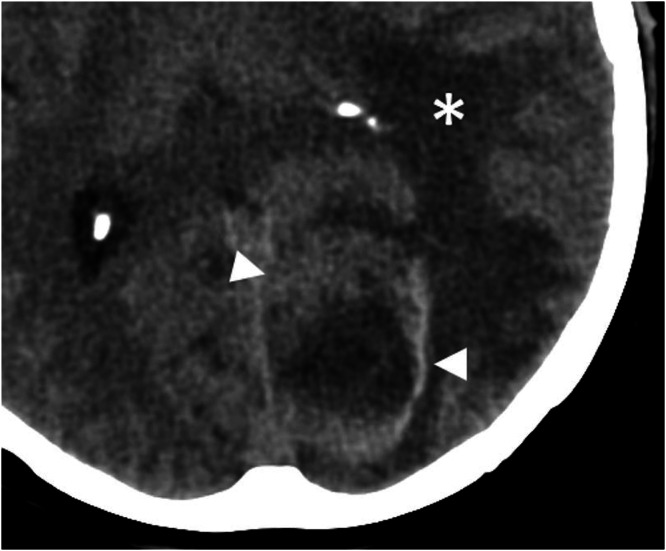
Pre-operative non-contrast CT with an axial reconstruction showing hypodense perilesional oedema (star) and hyperintense haemorrhage (arrowheads) surrounding an occipital lesion.
